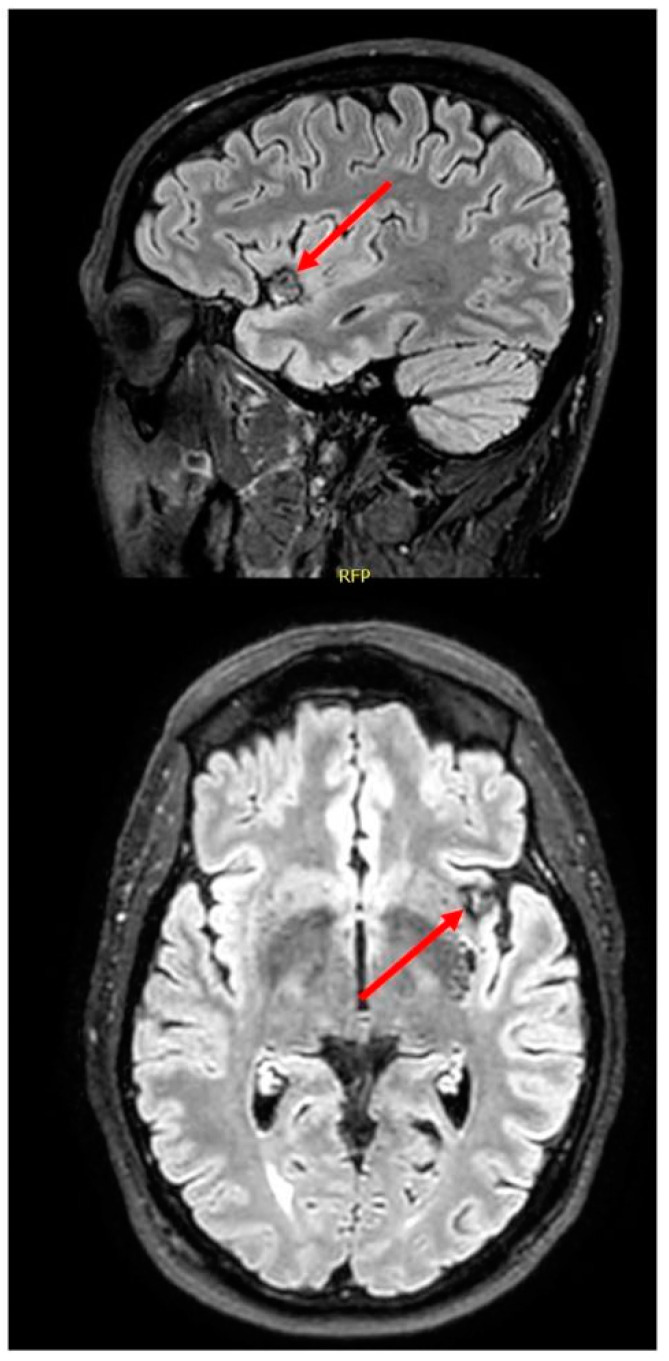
Brain magnetic resonance imaging (MRI) showing a left anterior insular cavernoma.
